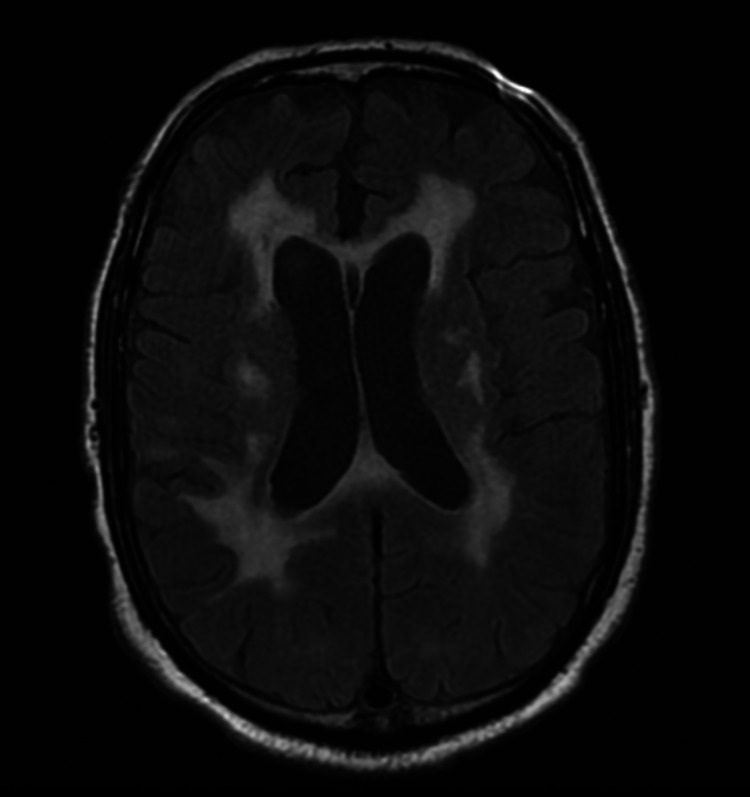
Repeat MRI in a year showing extensive abnormal FLAIR signal involving the bilateral cerebral hemispheres and no evidence of an abnormal enhancing intracranial mass. FLAIR, fluid-attenuated inversion recovery
